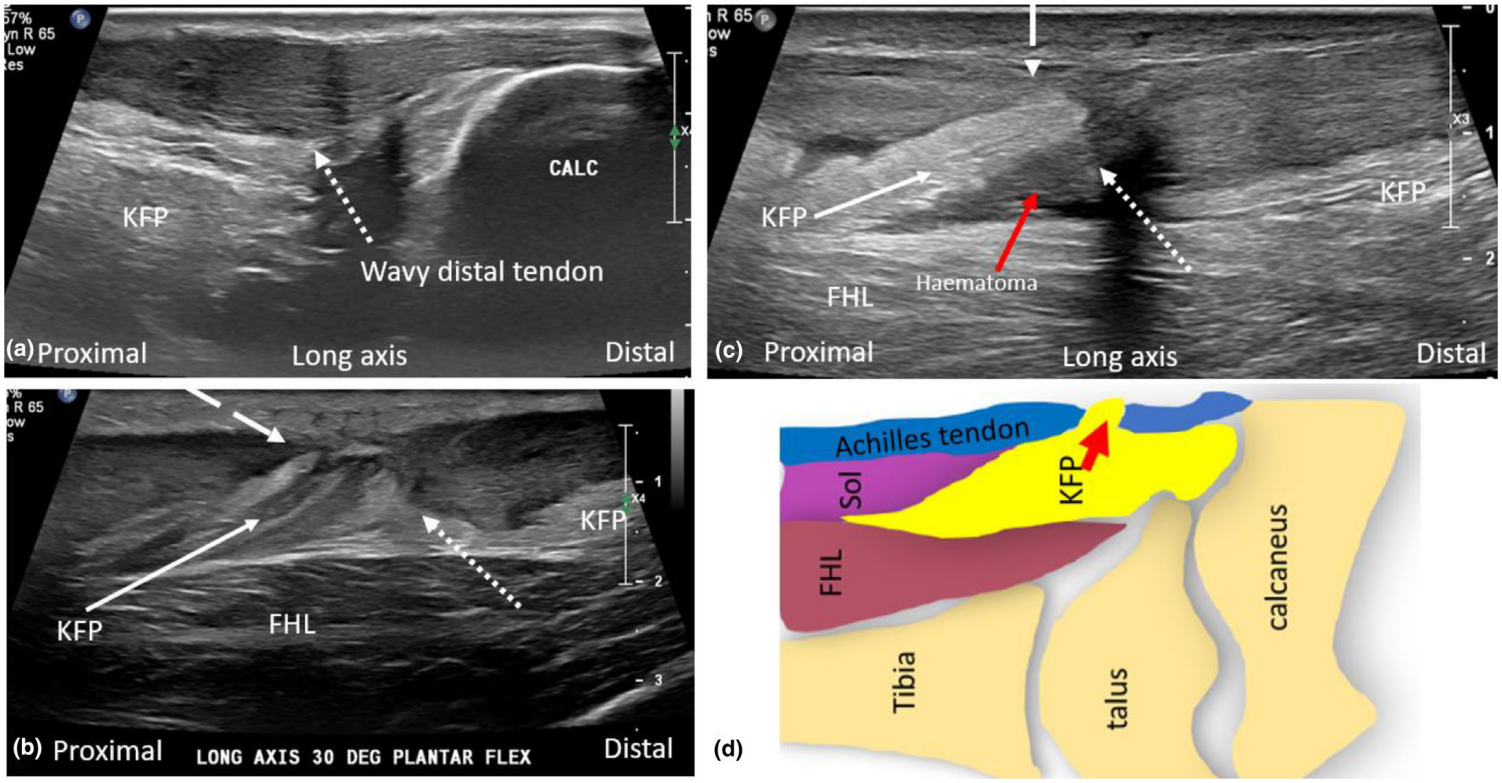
Long‐axis sonographic imaging of acutely ruptured Achilles tendon (mid tendon). (a) The tendon distal to the rupture (dotted arrow) appears wavy and not taut. (b, c) At the tendon rupture site, Kager's fat pad (KFP) can be seen herniating superficially and inferiorly through the gap between the proximal (dashed arrow) and distal (dotted arrow) tendon ends. (d) Depiction of the herniation of the KFP in a superficial and downward direction between ruptured Achilles tendon (blue) ends. Calc, calcaneus; FHL, Flexor hallucis longus muscle; KFP, Kager's fat pad; Sol, soleus muscle.
